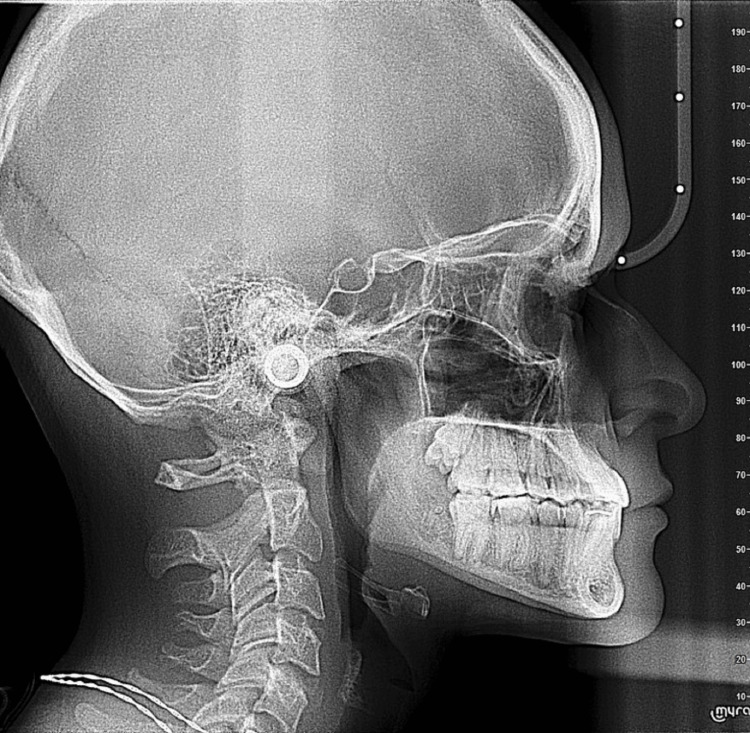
Post-treatment lateral cephalometric radiograph Lateral cephalometric radiograph obtained at the end of treatment, demonstrating a balanced facial profile, corrected incisor relationship, and stable skeletal relationships with minimal change in mandibular plane inclination.
